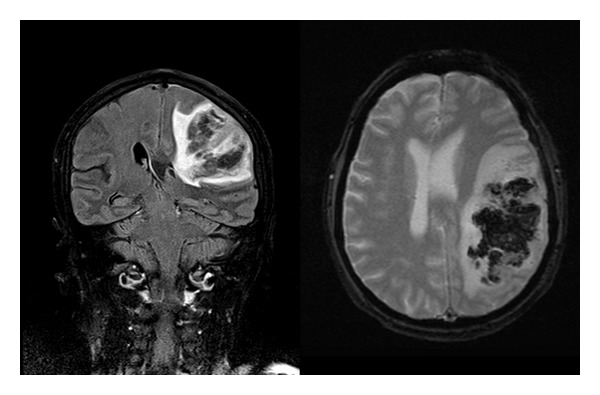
T2-weighted FLAIR magnetic resonance showing massive left frontotemporal hemorrhagic infarction.
